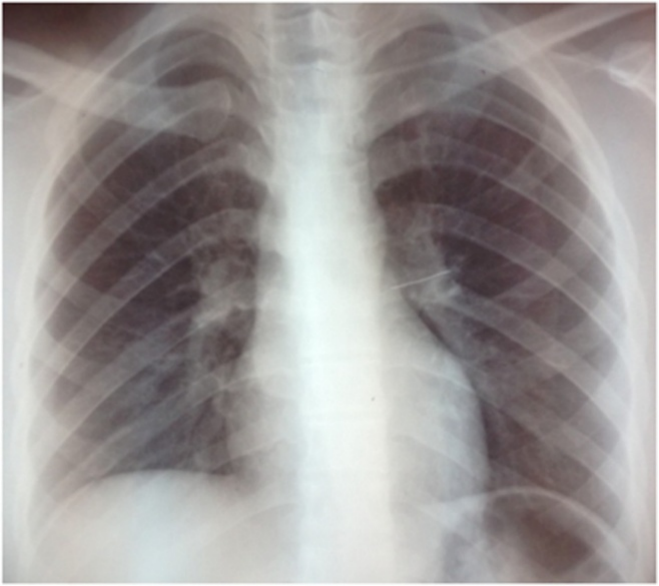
Chest radiograph showing a pin located in left superior lobar bronchus.
